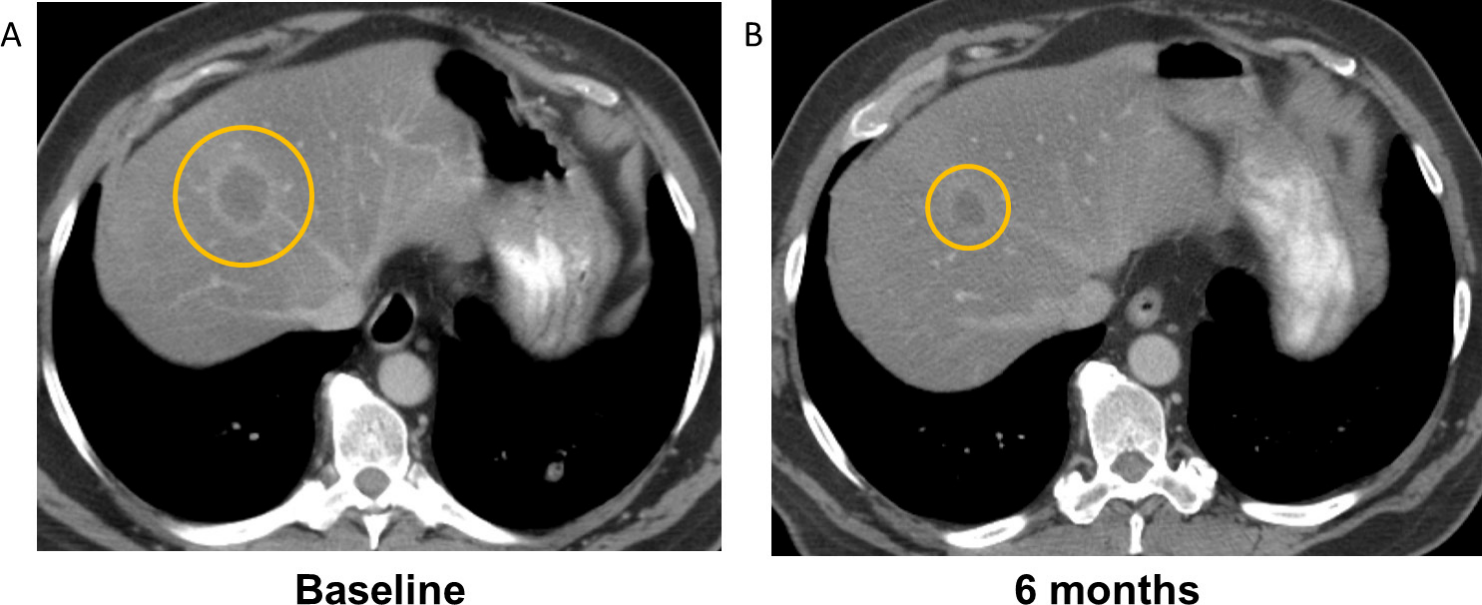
Computerized tomography (CT) demonstrating response to treatment with combination cetuximab, erlotinib, and bevacizumab in a patient with *KRAS* wild-type colorectal cancer who had received prior cetuximab and bevacizumab A decrease in tumor size of 45% by RECIST was observed, and the patient received treatment for 6 months. Panel A demonstrates a liver metastasis at baseline, and Panel B demonstrates the tumor after 6 months of treatment.
